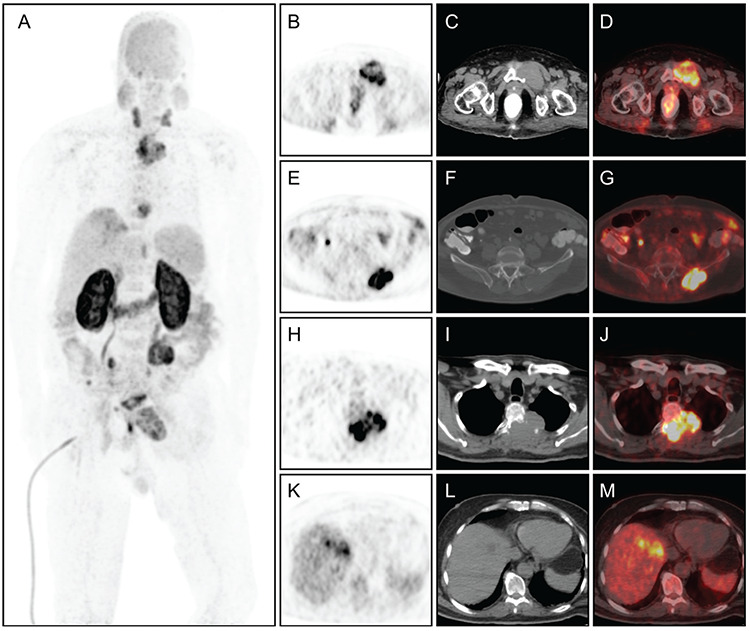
As an alternative PET/CT imaging, ^68^Ga-prostate-specific membrane antigen (PSMA) PET/CT was performed the same day. Corresponding to the lesions in ^18^F-FDG PET, ^68^Ga-PSMA PET/CT MIP (A), transaxial PET (B, E, H, K), CT (C, F, I, L), and fused (D, G, J, M) images demonstrated high PSMA expression in the metastatic bone lesions (SUV_max_=23.9) and in the primary liver tumor (SUV_max_=12.1). No other findings showed metastatic disease elsewhere in the body. PET/CT imaging with ^18^F-FDG has low diagnostic accuracy in assessing HCC patients because of its low metabolism (1). ^68^Ga-PSMA PET/CT is a new diagnostic technique to image recurrent prostate cancer (2). However, increased PSMA expression has been reported for different non-prostate malignancies, including HCC (3,4,5,6,7,8). There are only a few published documents on the merits of PSMA-PET for HCC (3,4,5,6,7,8). In fact, a few case reports and only 2 studies involving a small sample size has been reported in the recent past. In one of these studies, ^68^Ga-PSMA was reported to be superior relative to ^18^F-FDG for imaging HCC patients (7). However, in another study on advanced HCC patients, the PSMA expression was detected by ^68^Ga-PSMA PET, but it was not superior to that by ^18^F-FDG PET (8). In the current case, ^68^Ga-PSMA uptake was extremely high as compared to ^18^F-FDG uptake for bone metastases, and without ^18^F-FDG uptake in the primary tumor. Therefore, it is suggested that PET imaging with ^68^Ga-PSMA is helpful in HCC patients with low FDG affinity. Moreover, we believe that the existence of PSMA expression may act as a guide for radioligand therapy targeting PSMA in the future
